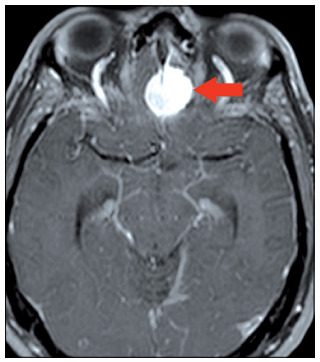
Axial contrasted T1-weighted magnetic resonance imaging exam showing olfactory groove meningioma
